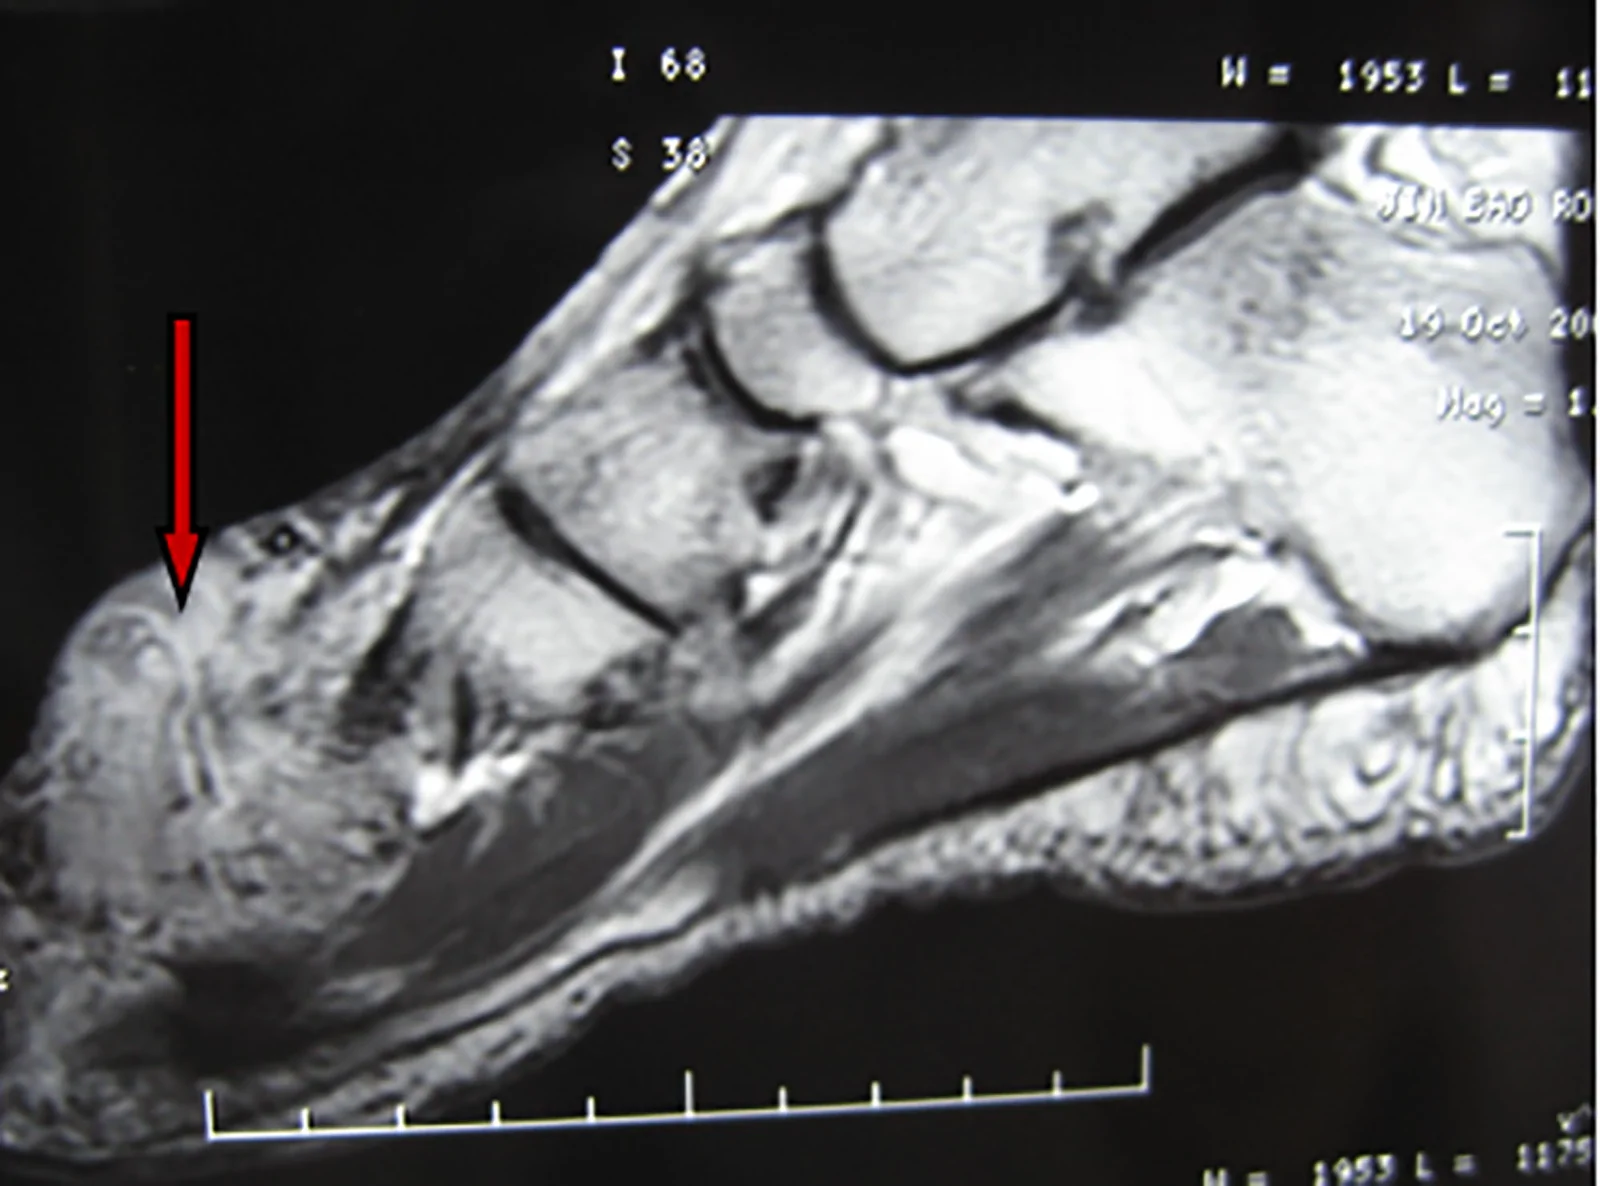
Enhanced magnetic resonance imaging (MRI) of the right foot revealing a soft tissue mass.
